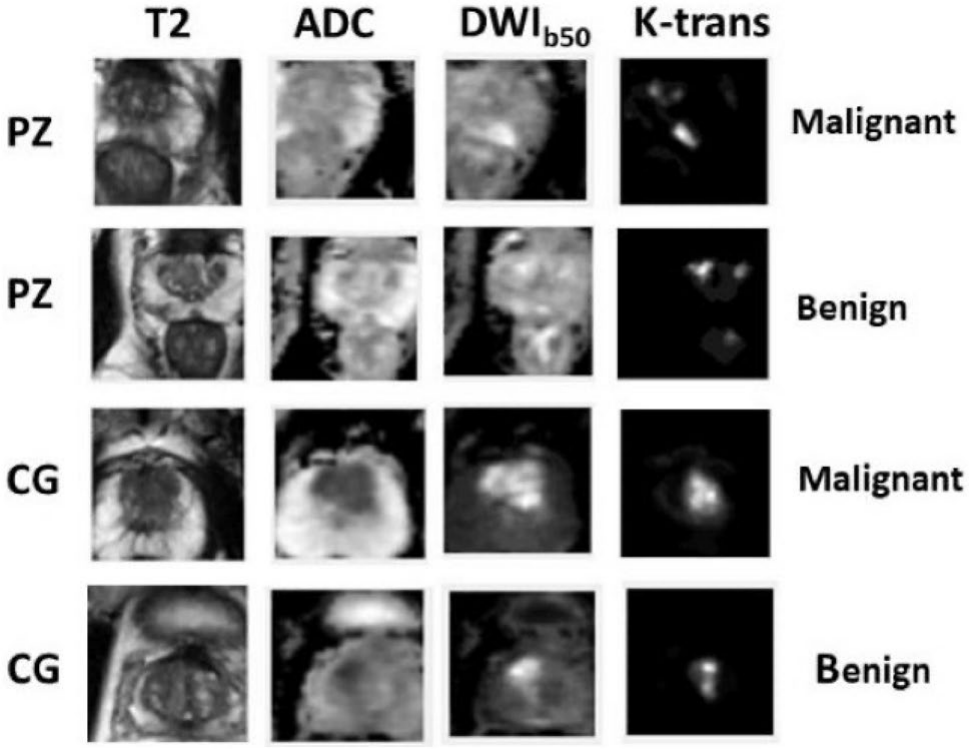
Sample images of the cropped 64 × 64 pixel rectangle from all four modalities: T2, ADC, DWI, and K-trans after resampling and registration. The lesions are malignant in PZ, benign in PZ, malignant in CG, and benign in CG from row 1 to 4 respectively.
